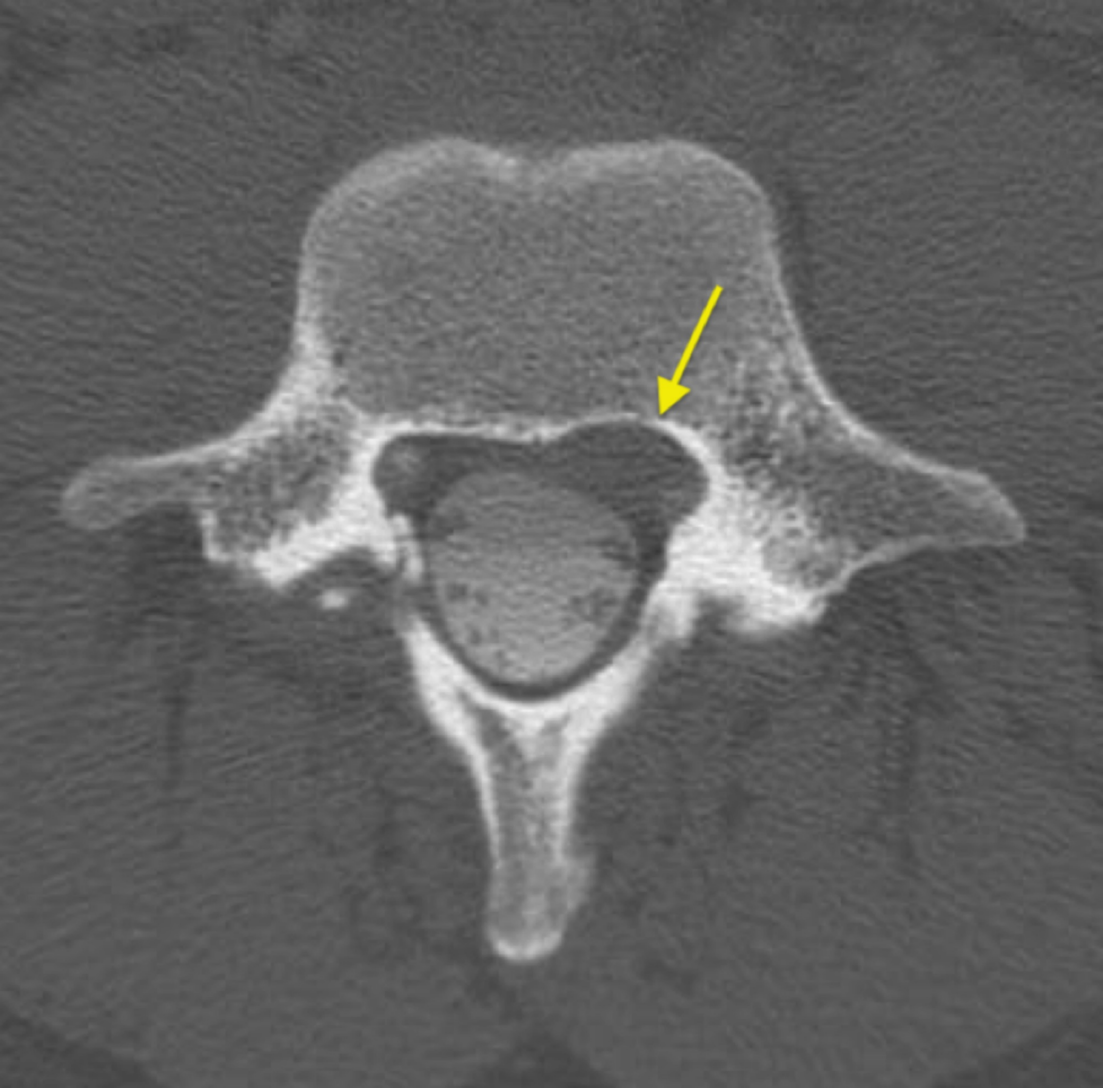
CT myelogram of the lumbar spine. Shows a left-sided cystic lesion with scalloping of the posterior vertebral body (arrow) with no contrast filling.
